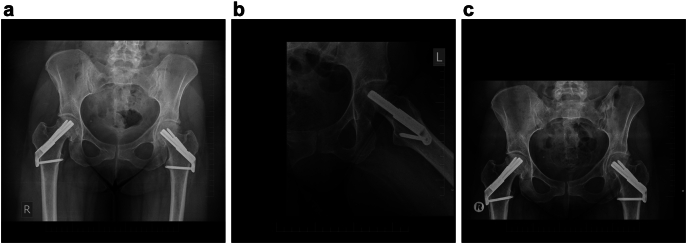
Radiographs of the affected hips at 3 and 6 months postoperatively. 4a., - Radiograph after bilateral femoral neck osteosynthesis, 3 months postoperatively- (AP) view. 4b, - Axial view 6 months postoperatively demonstrating partial avascular necrosis of the left femoral head. 4c, - Anteroposterior view 6 months postoperatively demonstrating partial avascular necrosis of the left femoral head.
